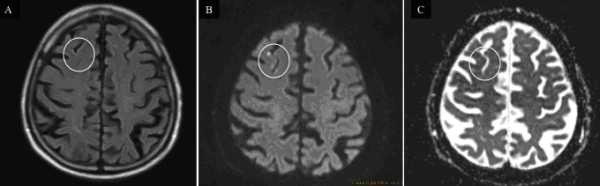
Post-ablation MRI with three distinct SCEs (white circle) not identified on FLAIR (A) but documented as hyperintense lesions in DWI (B) and corresponding hypointense lesion in ADC-map (C) defining SCE.
